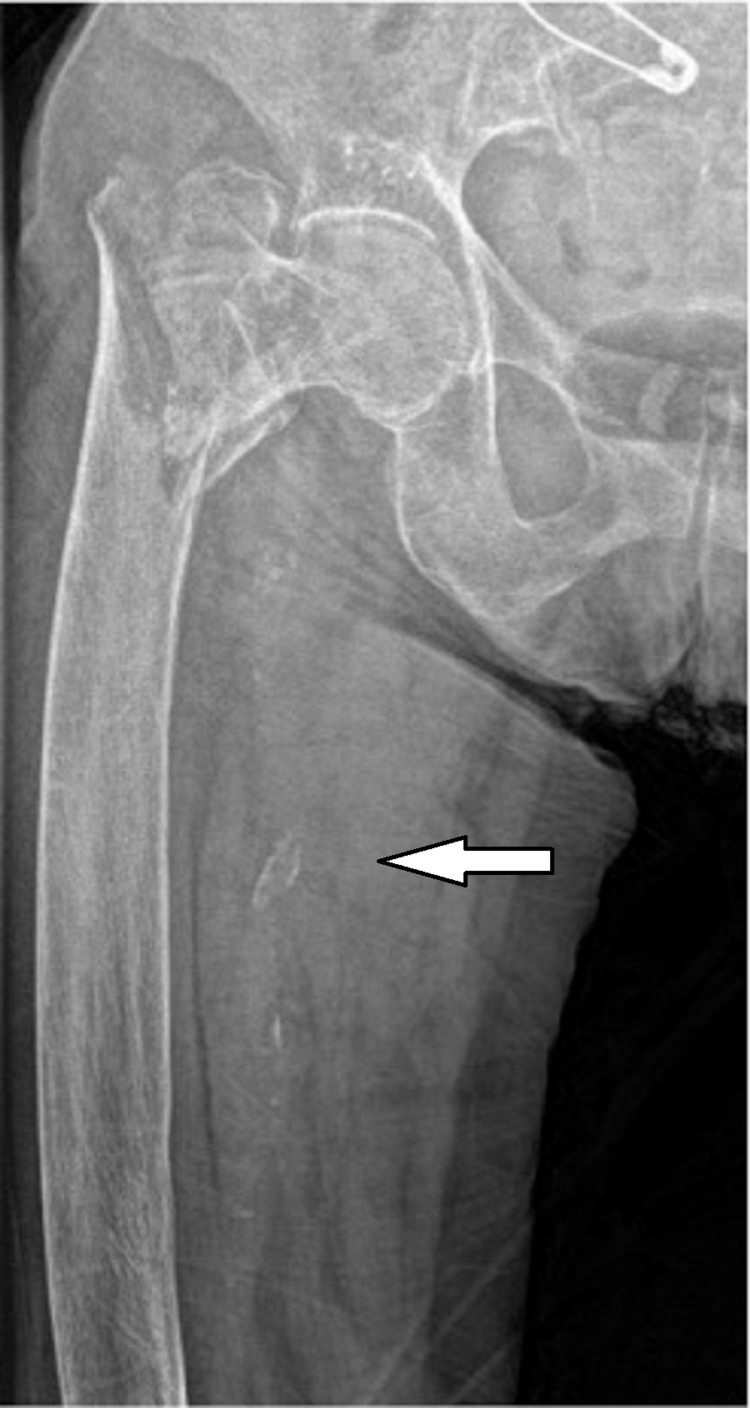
Radiographic image of the intimal type femoral artery calcification.
